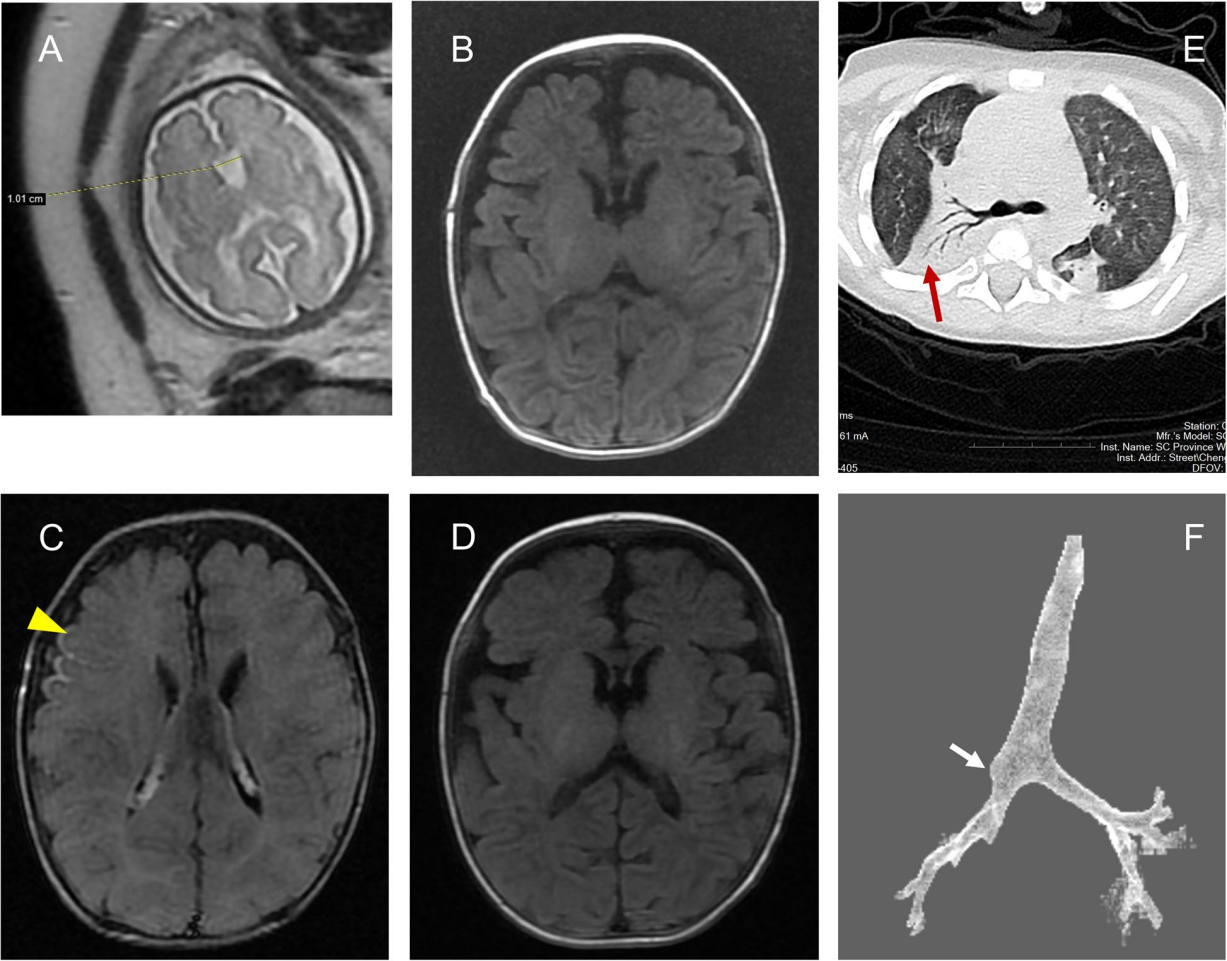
Brain MRI and chest CT images of the proband; A Fetal brain MRI at 30 + 6 weeks suggesting a slight widening of the Cavum septum pellucidum; B At 1 month and 30 days of birth, T1WI showed that high signals were faintly visible in the posterior parts of the hindlimbs of bilateral internal capsules; C At 1 month and 30 days old, T2-FLAIR delayed enhancement suggested right frontal pia enhancement, as indicated by the yellow arrow; D At the age of 3 months, T1WI showed high signals in the posterior limbs of bilateral internal capsules, but not in the forelimbs of internal capsules; E Chest CT indicates inflammation. Red arrows indicate lung consolidation; F 3D reconstruction of the airway showing a suspected stenosis of the bronchus of the right superior lobe indicated by the white arrow
